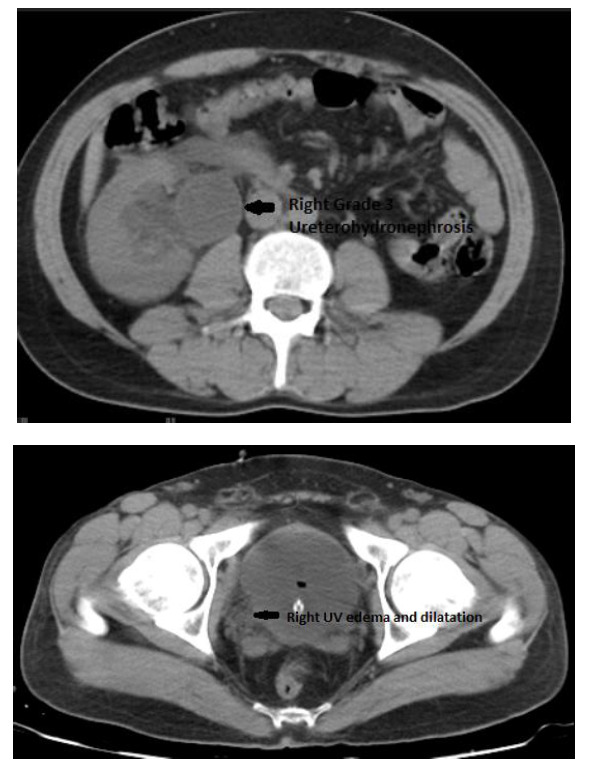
Abdomen CT image on the 2nd postoperative day, after D-J is withdrawn.
